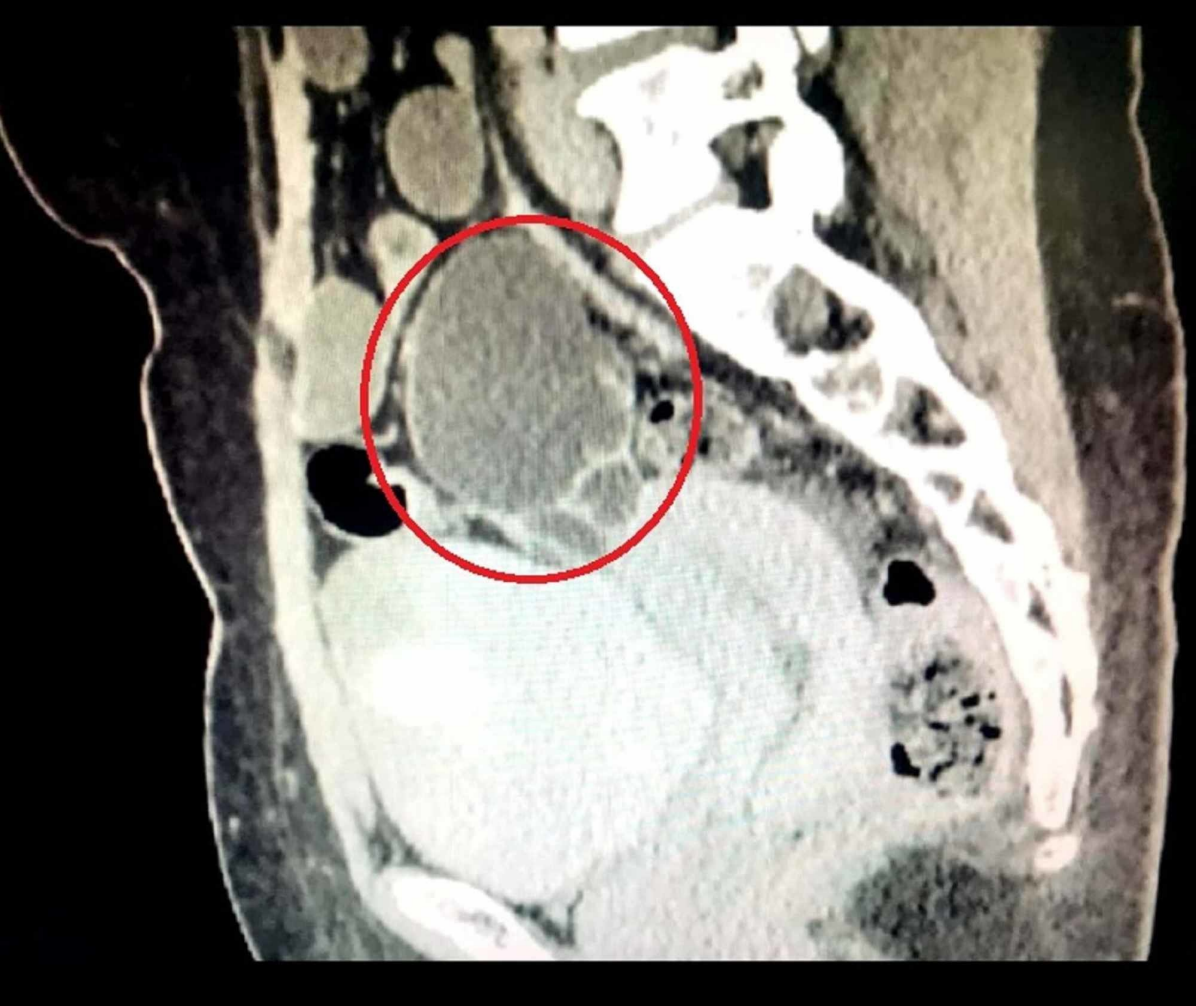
Sagittal CT scan of the abdomen/pelvis Sagittal CT depicting a large left adnexal mass (red circle)
